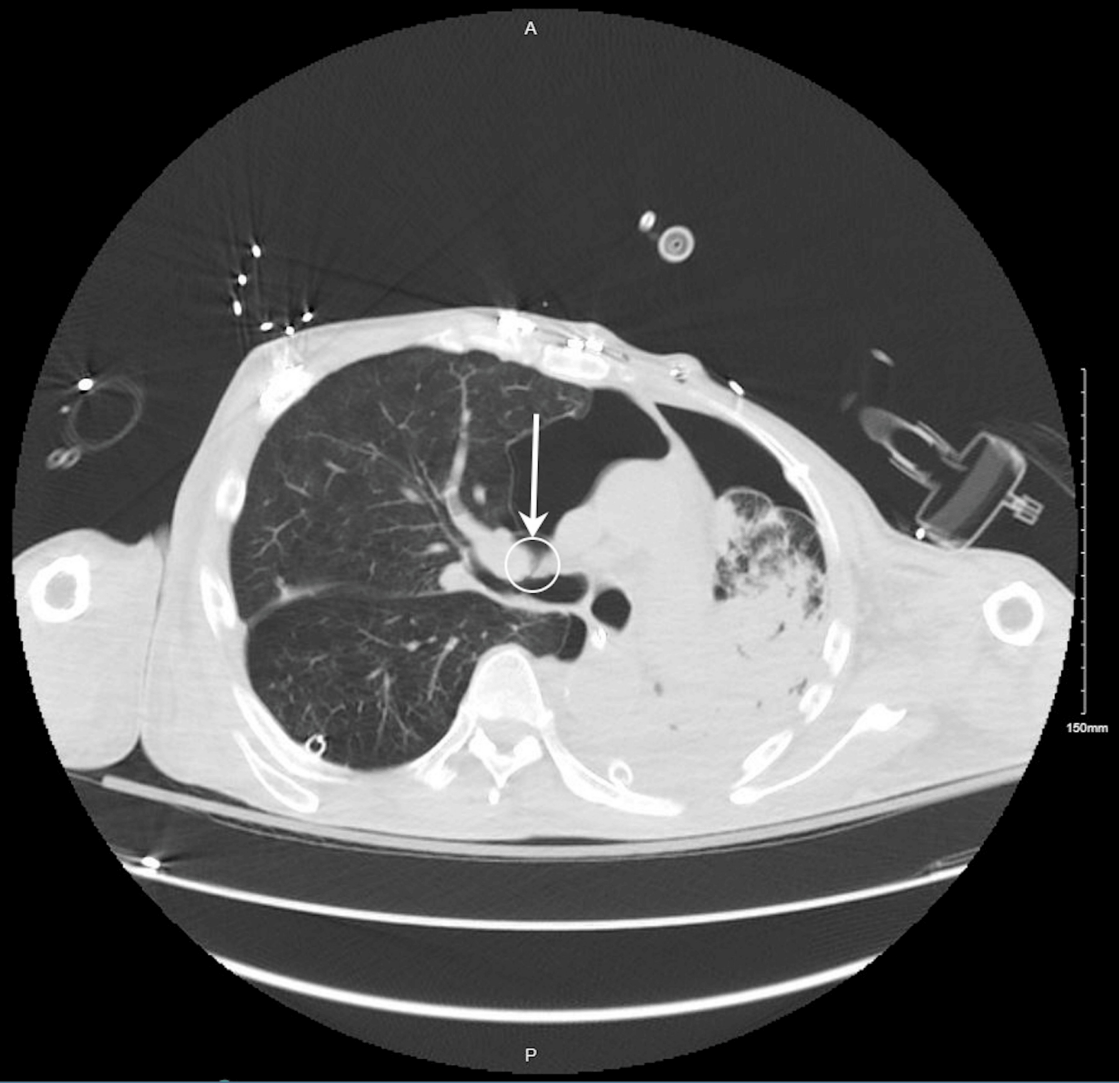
A repeat CT scan of the chest demonstrates a persistent large right and smaller left pneumothoraces with bilateral chest tubes in place. There is significant left lung consolidation/volume loss with left-shifting mediastinum. The broncho-pleural fistula is circled with arrows added.
